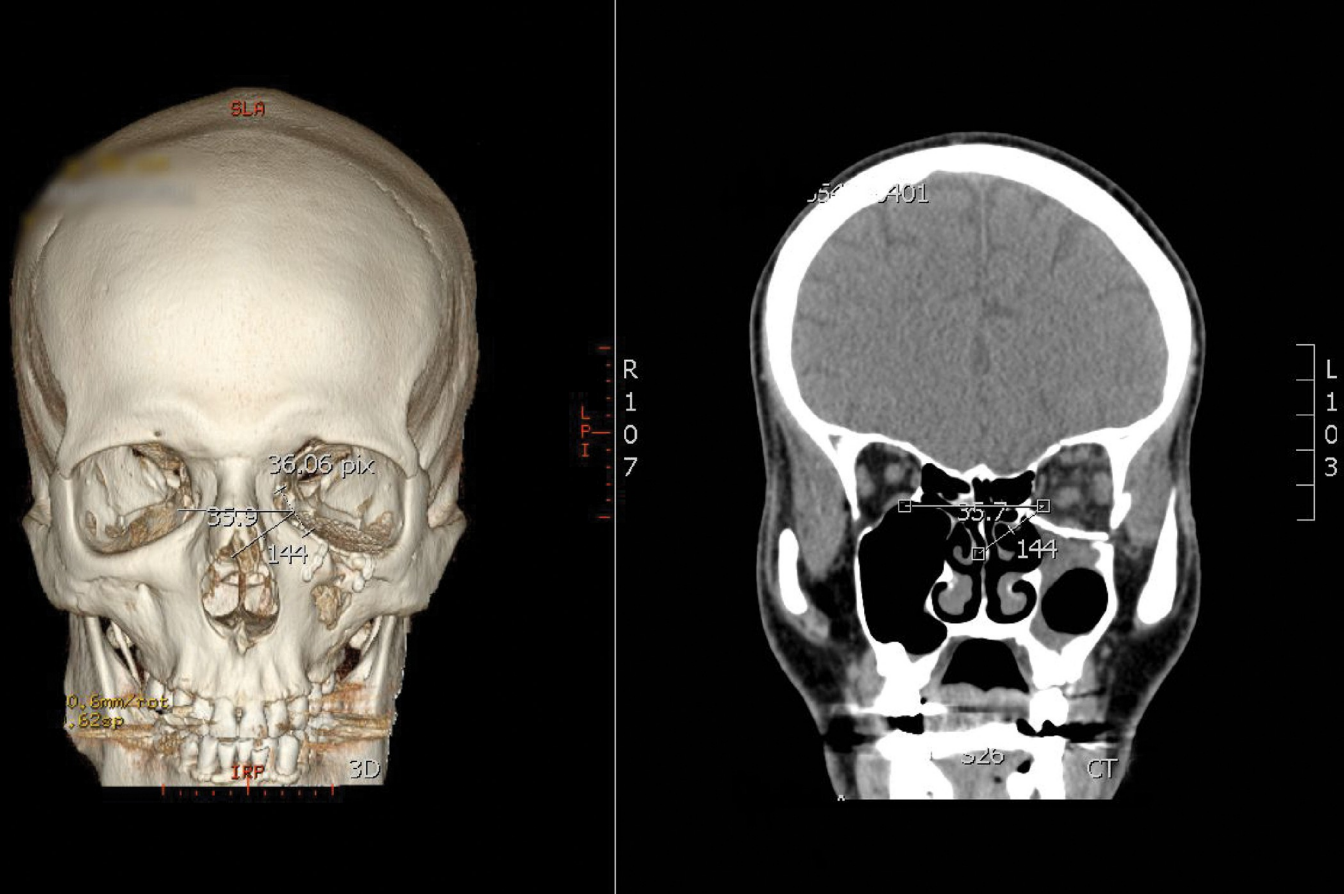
Shows a 3D reconstruction of a CT scan head in anteroposterior view (left) with the angle between the medial canthus and the middle turbinate measured on it and a coronal cut of the same CT scan head (right) with the angle measured on it (Khedr, Y).
